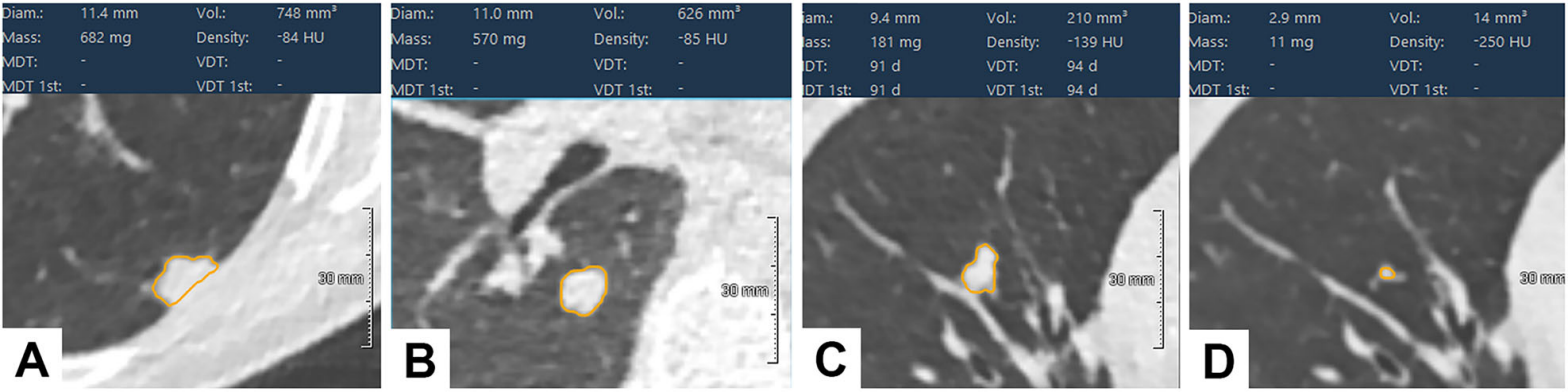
High-risk solid nodules. Axial LDCT images from different patients. A, B correspond to two different patients, and C, D correspond to a third patient with two studies. A A pleural-based solid nodule in the left lower lobe, with a volume of 748 mm^3^ at baseline. Positive FDG-PET led to a percutaneous biopsy confirming malignancy. A pT2 lung adenocarcinoma invading the visceral pleura was resected. B A 626 mm^3^ solid nodule in the right Nelson segment was found at baseline. The nodule was negative on PET and remained stable on follow-up CT scan. C A solid nodule of 210 mm^3^ in the middle lobe was discovered at one year follow-up, which already existed on the baseline scan (D). VDT was only 94 days. An 11 mm squamous cell carcinoma was found at the time of surgery
